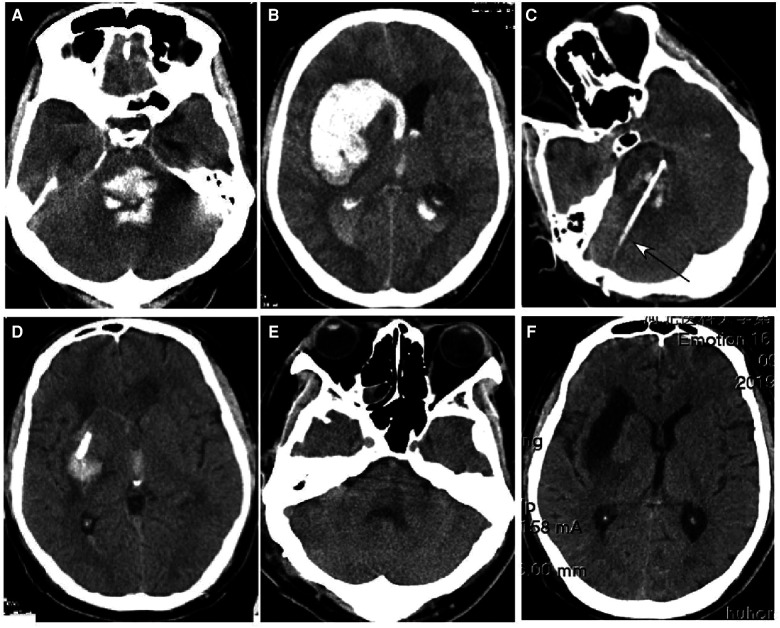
A 39-year-old man had a sudden onset of unconsciousness 3 h before presentation. (A,B) A head CT scan showed severe hemorrhage in the brainstem (A), basal ganglia, and lateral ventricle (B). The patient was in a coma with no spontaneous breathing and with a Glasgow Coma Scale (GCS) score of 3 (E1VTM2). (C,D) An ultra-early stereotactic aspiration was performed (arrow). After surgery, the hemorrhage greatly reduced, and the patient regained his spontaneous breathing with a GCS score of 4 (E1VTM3). (E,F) One month later, the hemorrhage had completely disappeared.
